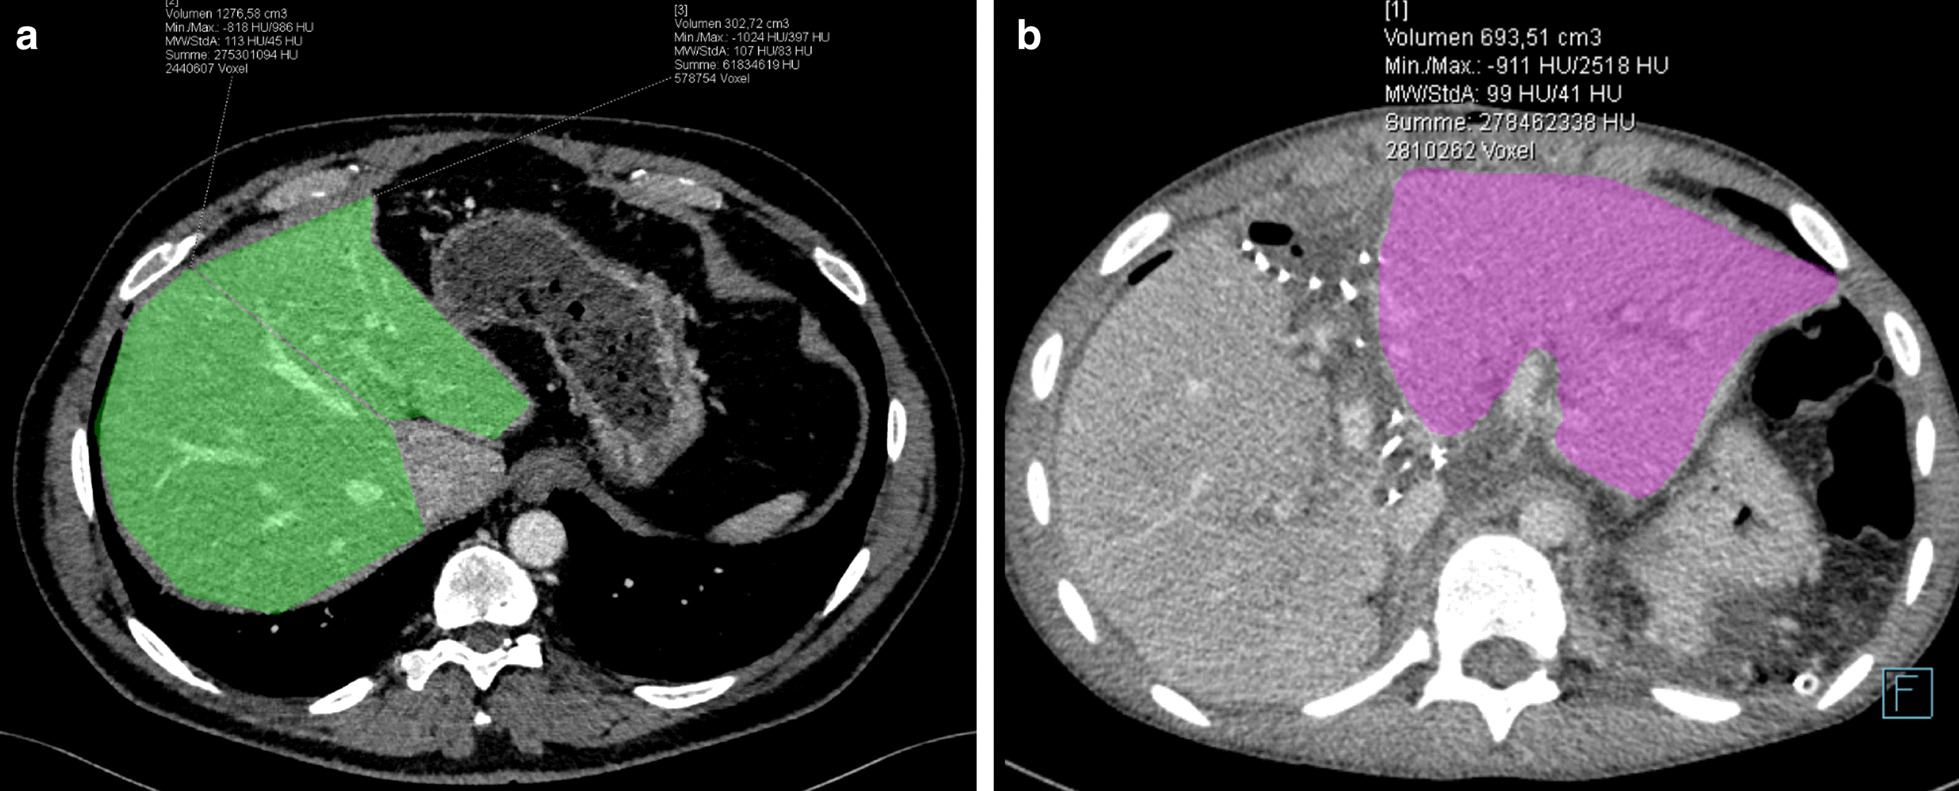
Preoperative CT scan of donor 2. a Volumetry of liver segment II/III (303 cm^3^) and segments V–VIII (1277 cm^3^). b CT scan of recipient 2 at postoperative day 10 (before removal of the native right liver) with volumetry of the graft (694 cm^3^) after hypertrophy
